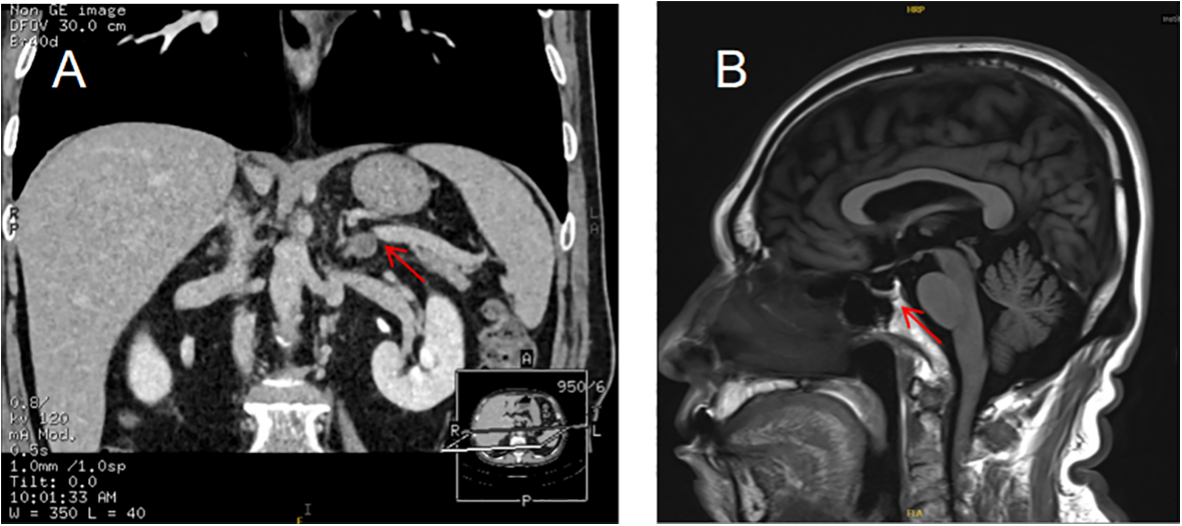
Case 2: Contrast-enhanced 3D-CT of the adrenal glands showed an oval nodule at the junction of the left adrenal gland, measuring 1.2×1.0 cm (A). Cranial magnetic resonance imaging (MRI) showed empty sella turcica of the pituitary gland (B).
